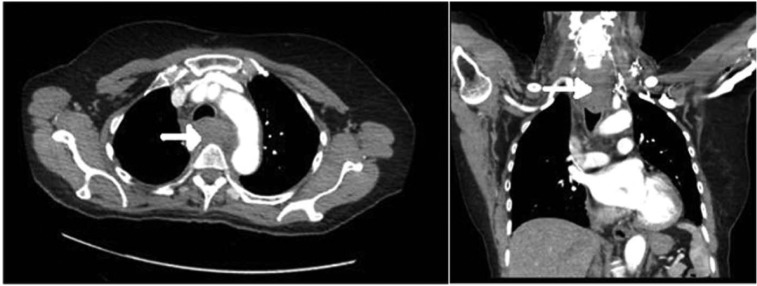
CT thorax with contrast at presentation. The hematoma can be seen causing mediastinal compression.
